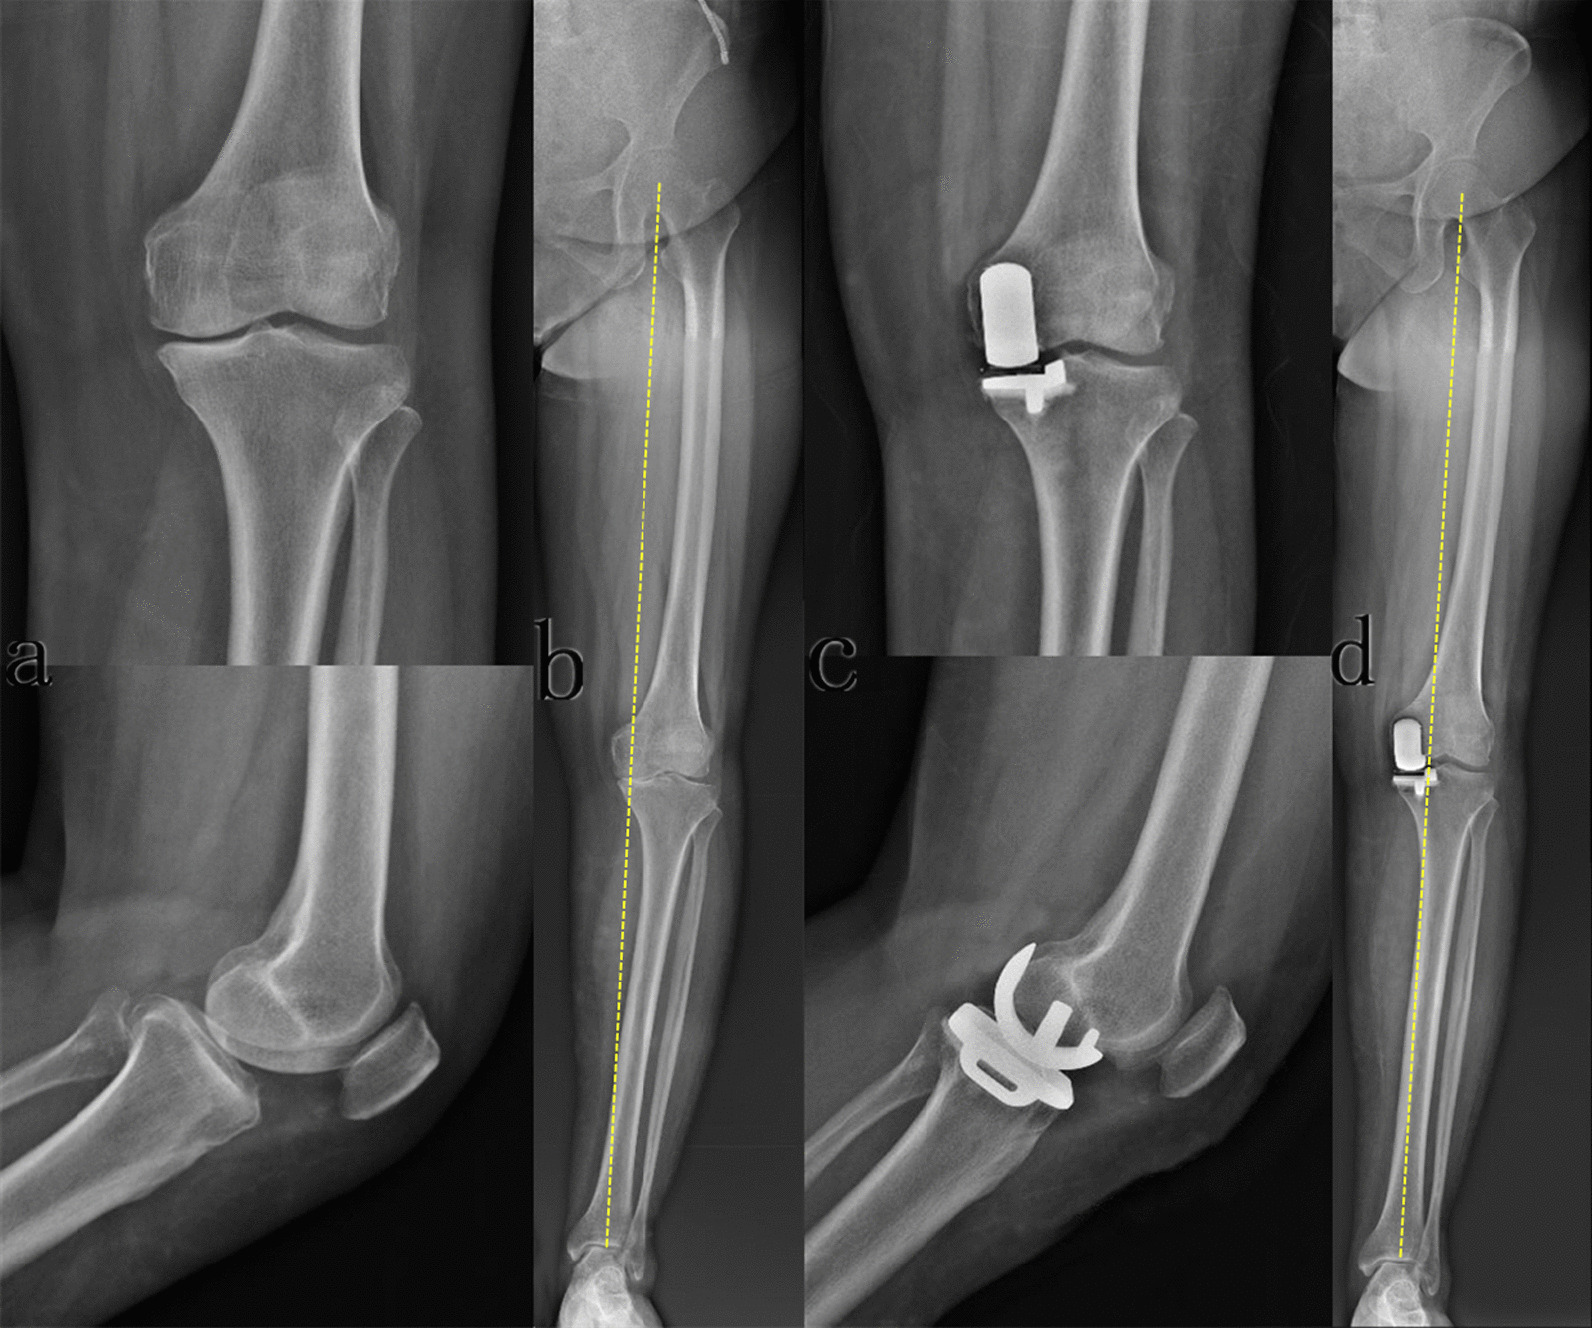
Representative images of mobile-bearing UKA. a Preoperative X-ray scans at the anteroposterior and lateral views suggested anteromedial osteoarthritis of the knee joint; b preoperative full-length standing anteroposterior radiographs suggested bone-to-bone contact in the medial compartment, with varus angles of 7.96°; c postoperative X-ray scans at the anteroposterior and lateral views suggested acceptable placement of the prosthesis; d postoperative full-length standing anteroposterior radiographs suggested acceptable placement of the prosthesis, with varus angles of 3.71°
